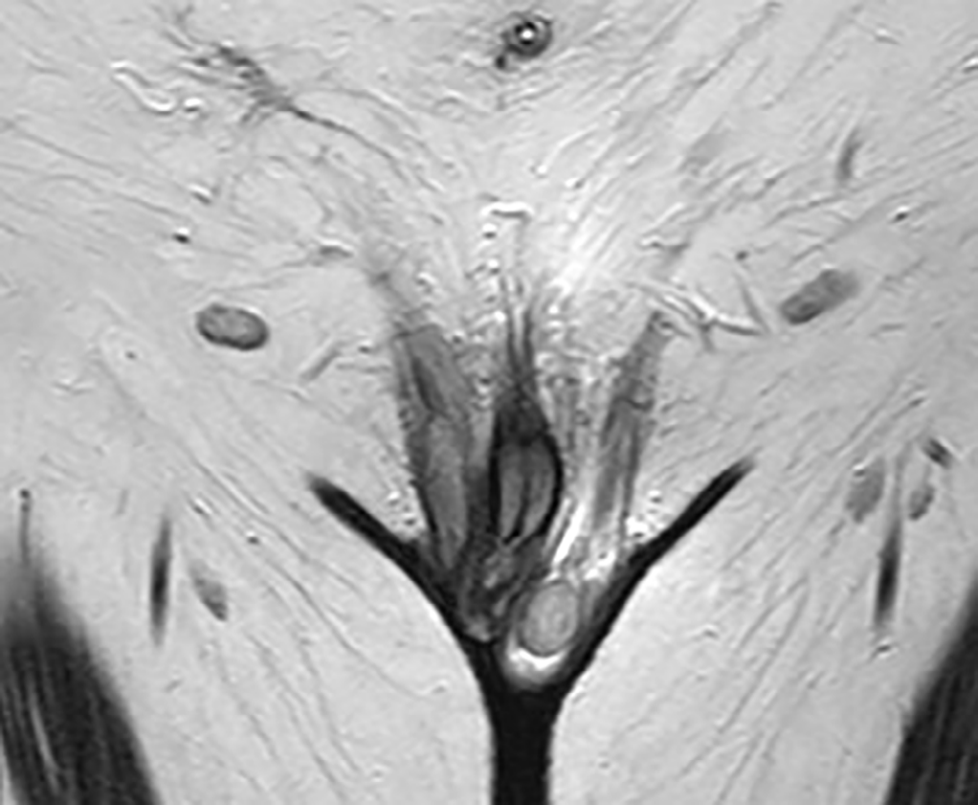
T2-weighted coronal image: hypertrophic clitoris with cavernosa corpora
